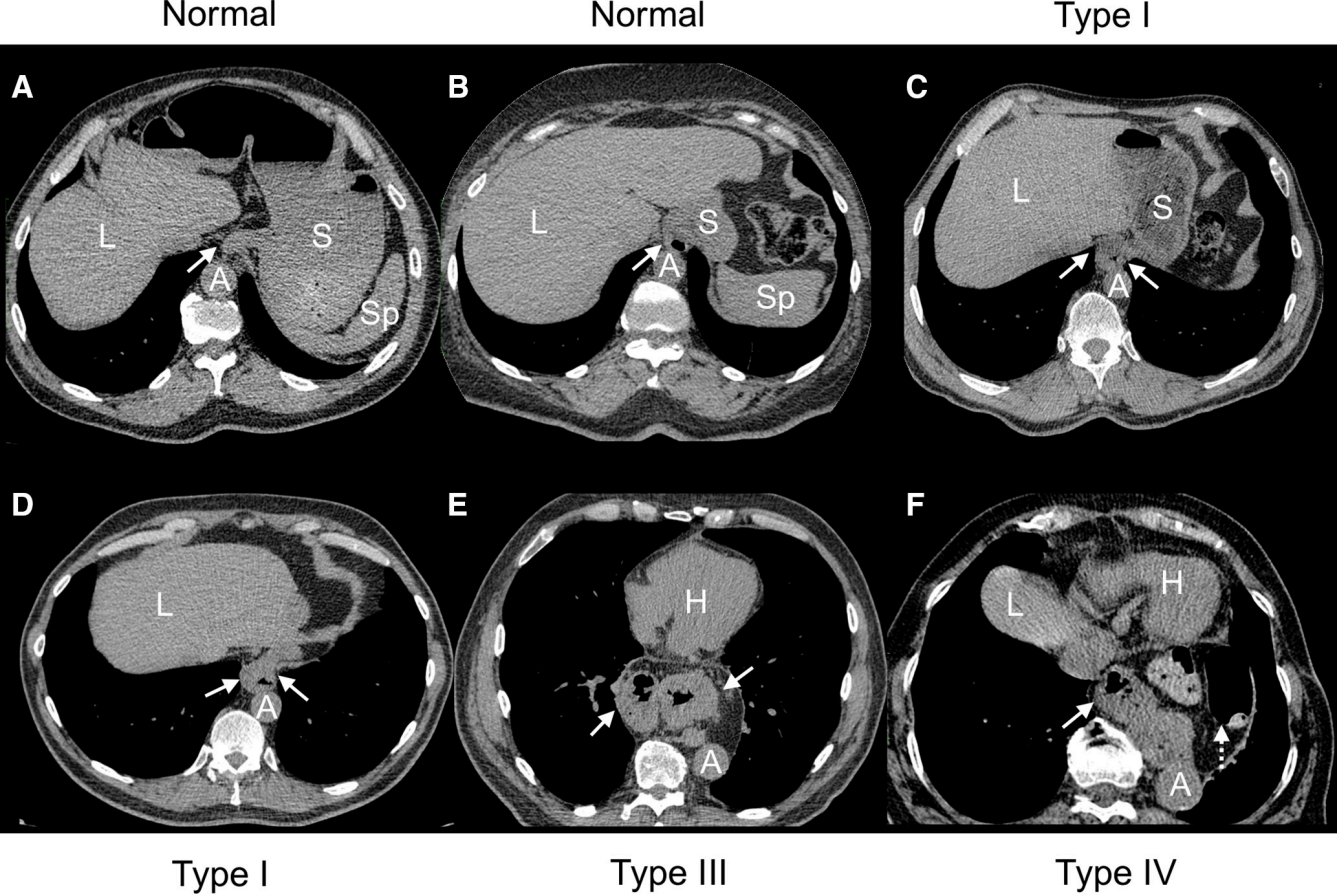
Examples of normal gastro-oesophageal (GE) junction and types I, III and IV hiatal hernia (HH) on non-contrast CT. (A) Non-contrast chest CT of a man aged 80 years with three reviewers reporting normal GE junction (arrow). (B) Non-contrast chest CT of a woman aged 59 years with type 1 HH (arrow) reported by one of three reviewers and considered normal by the other two reviewers. (C) Non-contrast chest CT of a man aged 85 years with two out of the three reviewers reporting type I HH (arrows). (D) Non-contrast chest CT of a man aged 64 years with all three reviewers reporting type I HH (arrows). (E) Non-contrast chest CT of a man aged 75 years with three reviewers reporting type III HH (arrows). (F) Non-contrast chest CT of a woman aged 84 years with three reviewers reporting type IV HH (solid arrow) with colon in the thorax (dashed arrow). A, aorta; H, heart; L, liver; S, stomach; Sp, spleen.
